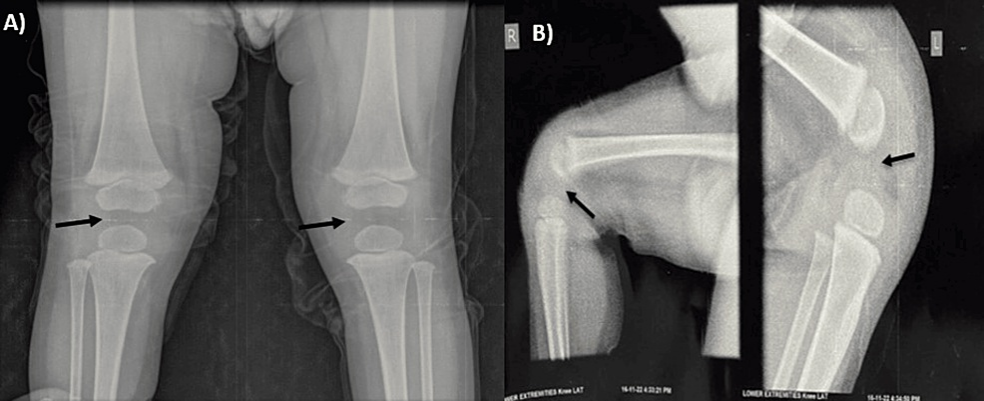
X-ray image confirming the absence of the patella Antero-posterior (A) and lateral (B) radiograph of bilateral knee. Arrows indicate the absence of the patella in the anterior aspect of the knee joint.
